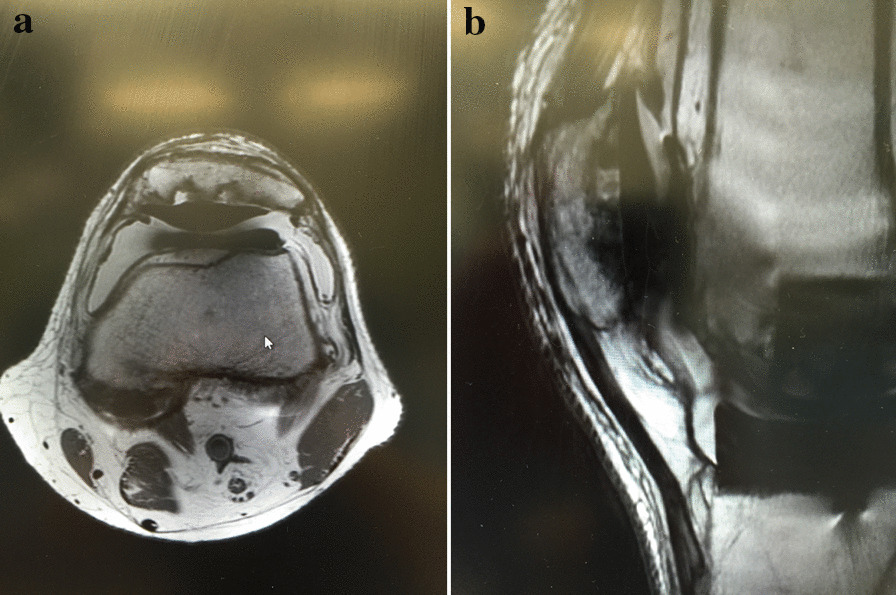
a Patella loosening on preoperative MRI transversal images of a 55-year-old male patient undergoing patella revision. b Sagittal MRI images of the same patient
